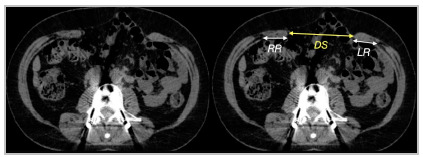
CT image showing the relationship between the measurement of the defect (DS) and the rectus muscles (RR and RL) to assess any need for separation of chemical or surgical components.
